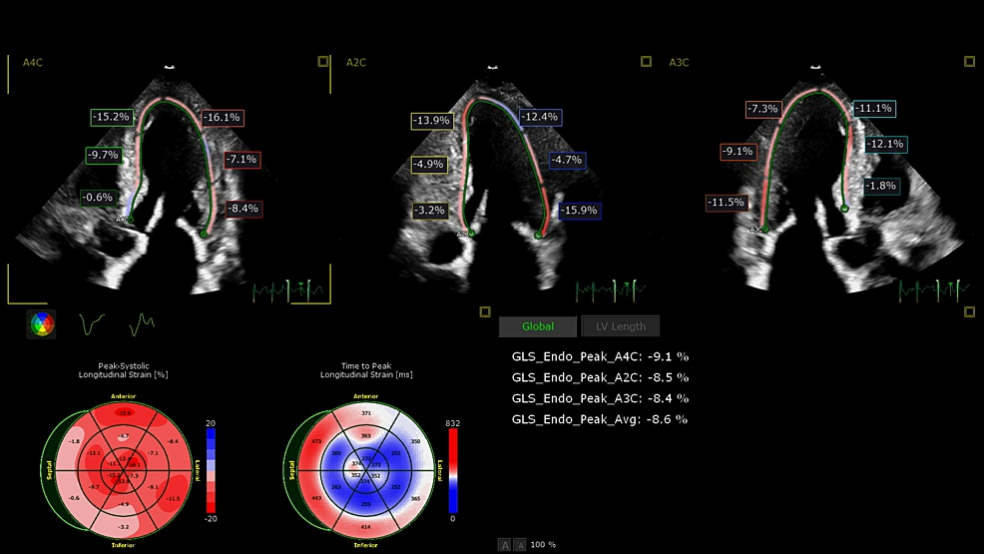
Echocardiogram results indicating left ventricular strain associated with amyloidosis and sub-clinical left ventricular dysfunction.
